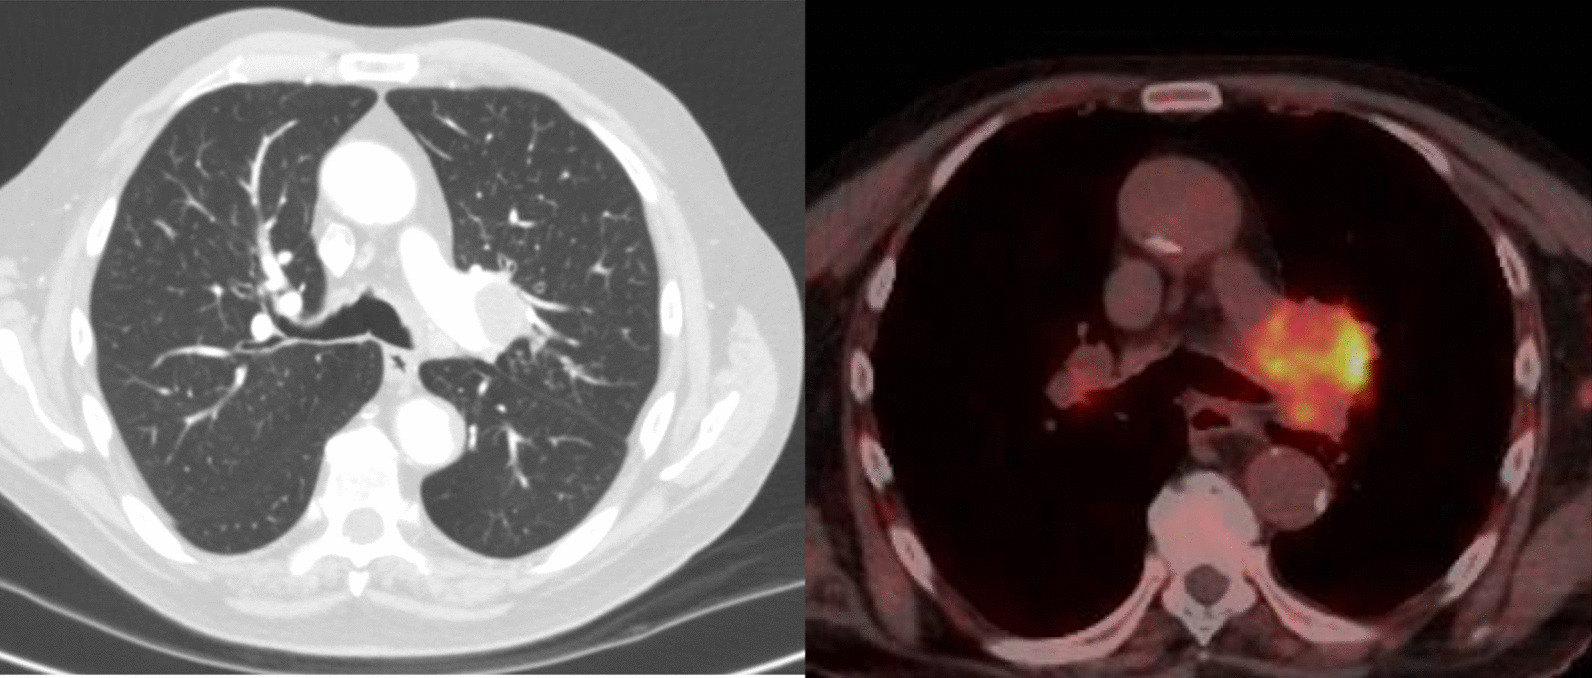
Chest CT showing left mediastinal lymphadenopathy (image A) with associated PET avid lung lesion (image B)
